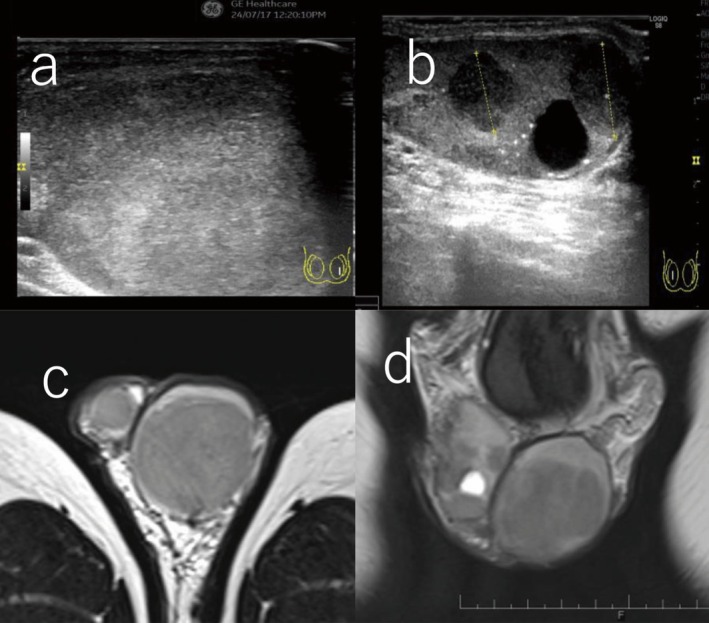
MRI and US of bilateral testes after 5 years: (a) The left testis was entirely mosaic‐like and replaced by a neoplastic lesion. (b) Low echoing areas 9.7 mm and 11.1 mm in diameter were observed on a cystic nodular lesion. (c, d) MRI showed a low‐signal neoplastic lesion in the left testis on T2‐weighted imaging, and a similar neoplastic lesion around a cystic lesion in the right testis.
